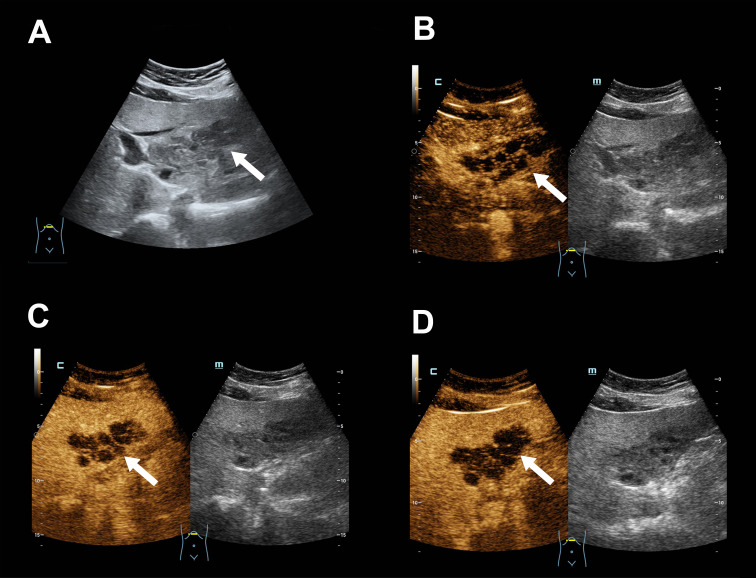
Liver contrast-enhanced ultrasound (CEUS) imaging. (A) A hypoechoic mass with indistinct margins, irregular contour, and heterogeneous internal echoes in the left lateral lobe of the liver. (B) Arterial phase (12 seconds): sparse punctate-linear enhancement within the mass, appearing isoenhancing. (C) Portal phase (69 seconds): the mass shows slight hypoenhancement. (D) Late parenchymal phase (136 seconds): the mass remains slightly hypoenhancing, with most of the lesion lacking enhancement and exhibiting a tunnel-like appearance. (The mass is indicated by white arrows in all panels.).
